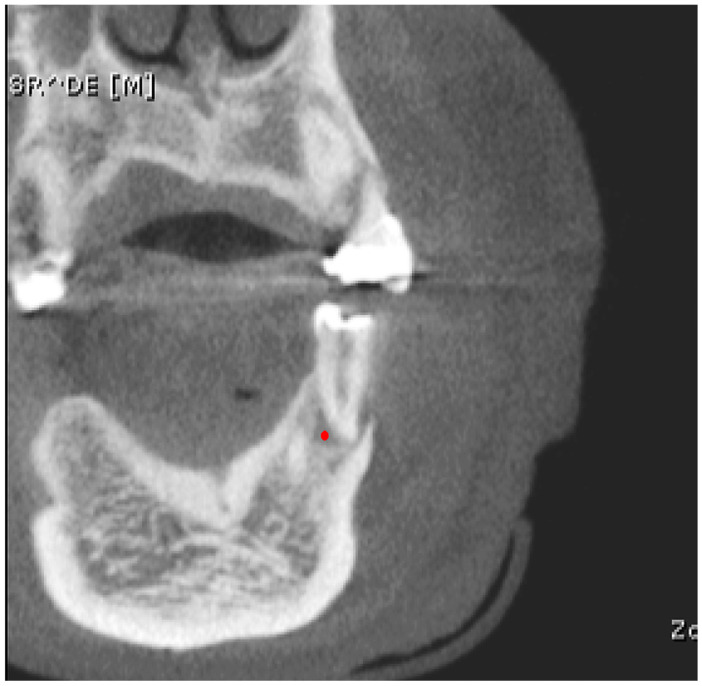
CBCT slice demonstrating the placement of the circular region of interest (ROI) for texture analysis of periapical lesions using MaZda software. The red dot indicates the center of the periapical lesion, determined at the intersection of the lateromedial and superoinferior lines. A circular ROI with a diameter of 44 pixels was centered on this point to ensure only lesion tissue was included in the analysis.
